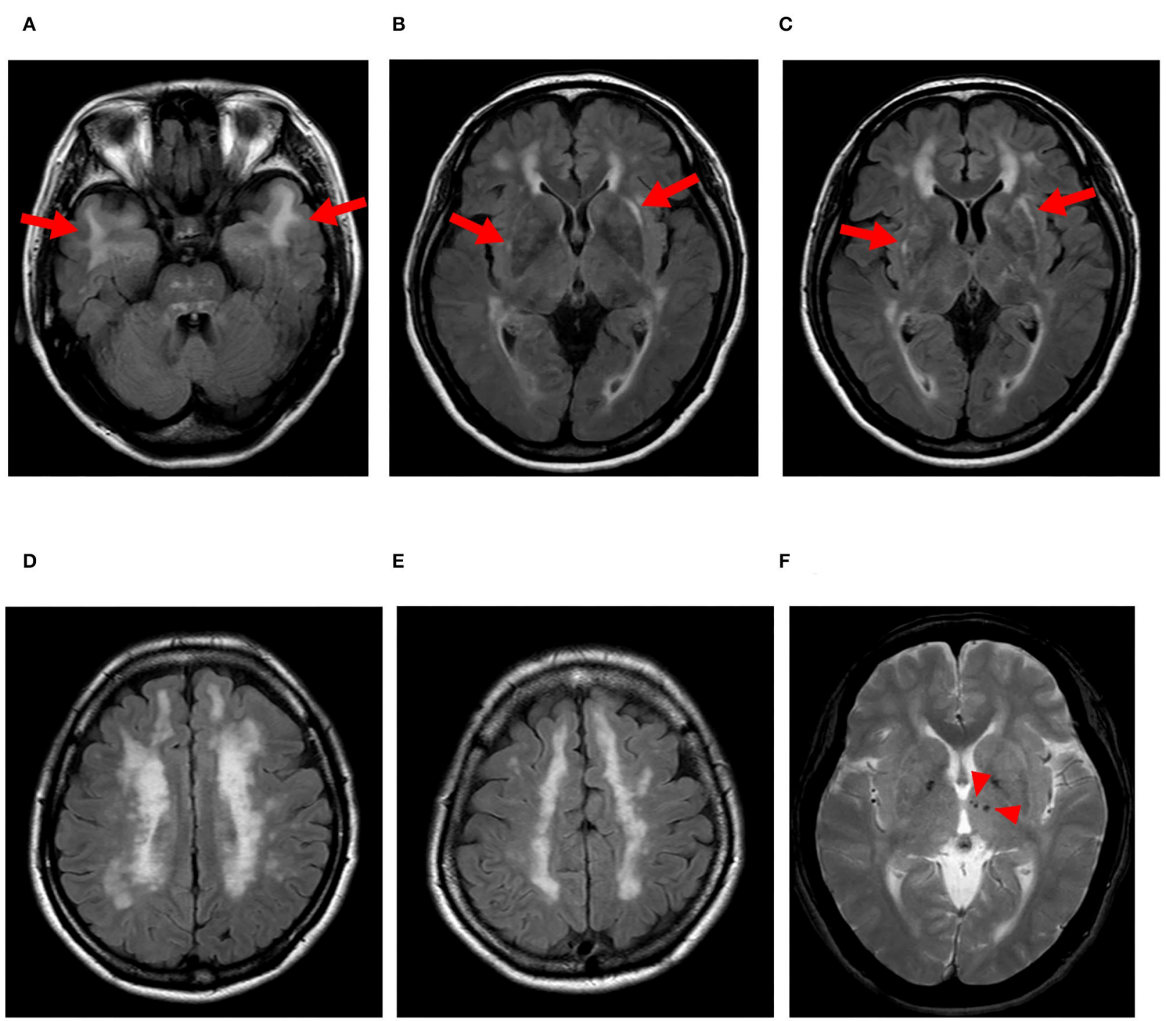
Representative MRI findings of CADASIL. Case 2 with the pathogenic *NOTCH3* p.Arg110Cys variant. (A–E) FLAIR images, (F) T2-star weighted image. (A) Arrows indicate lesions in the temporal pole. (B,C) Arrows indicate lesions in the extra capsule of the putamen, and (F) arrowheads indicate microbleeds.
